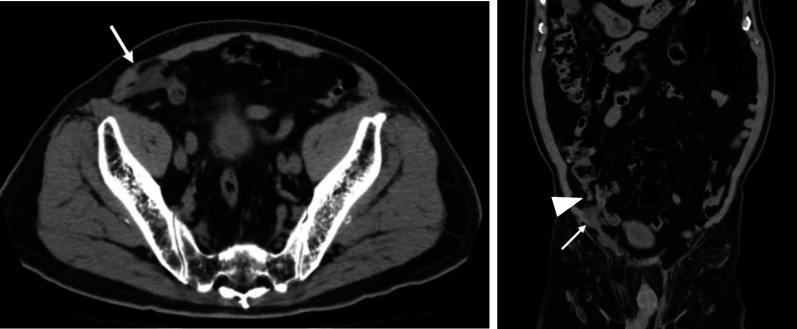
Computed tomography (CT) findings 6 years prior to surgery. 2–1 The CT scan shows fluid around the plug mesh, which was considered to be the cause of mild inflammation (arrow). 2–2 The CT scan shows that the mesh (arrow) and appendix (arrowhead) were not in contact
